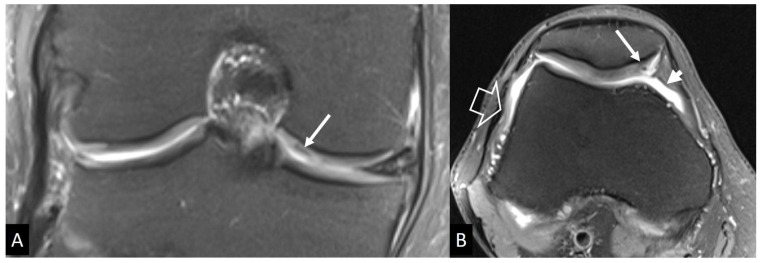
A 62-year-old athletic male, with severe joint pain, warmth, and swelling. The final diagnosis was CPPD arthritis. Coronal (A) and axial (B) fat-suppressed PD-w MR images showing mild effusion (short arrow). Synovitis (open arrow) and chondrocalcinosis (thin arrows) suggest CPPD.
